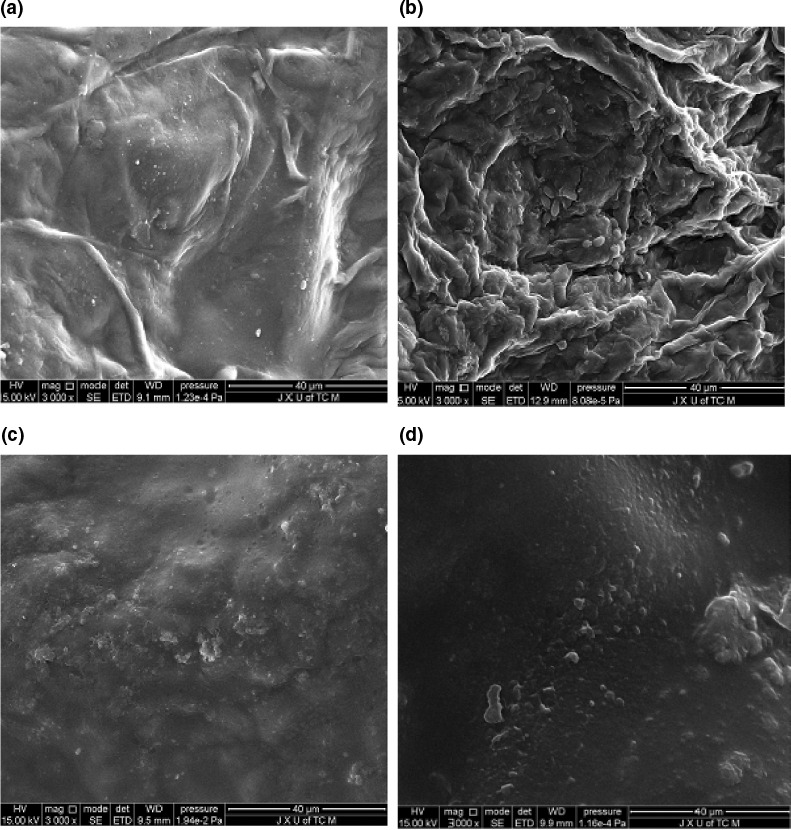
SEM images of (a) raw material, and residues obtained by (b) hydrodistillation extraction; (c) ultrasound‐assisted hydrodistillation extraction; (d) microwave‐assisted hydrodistillation extraction
